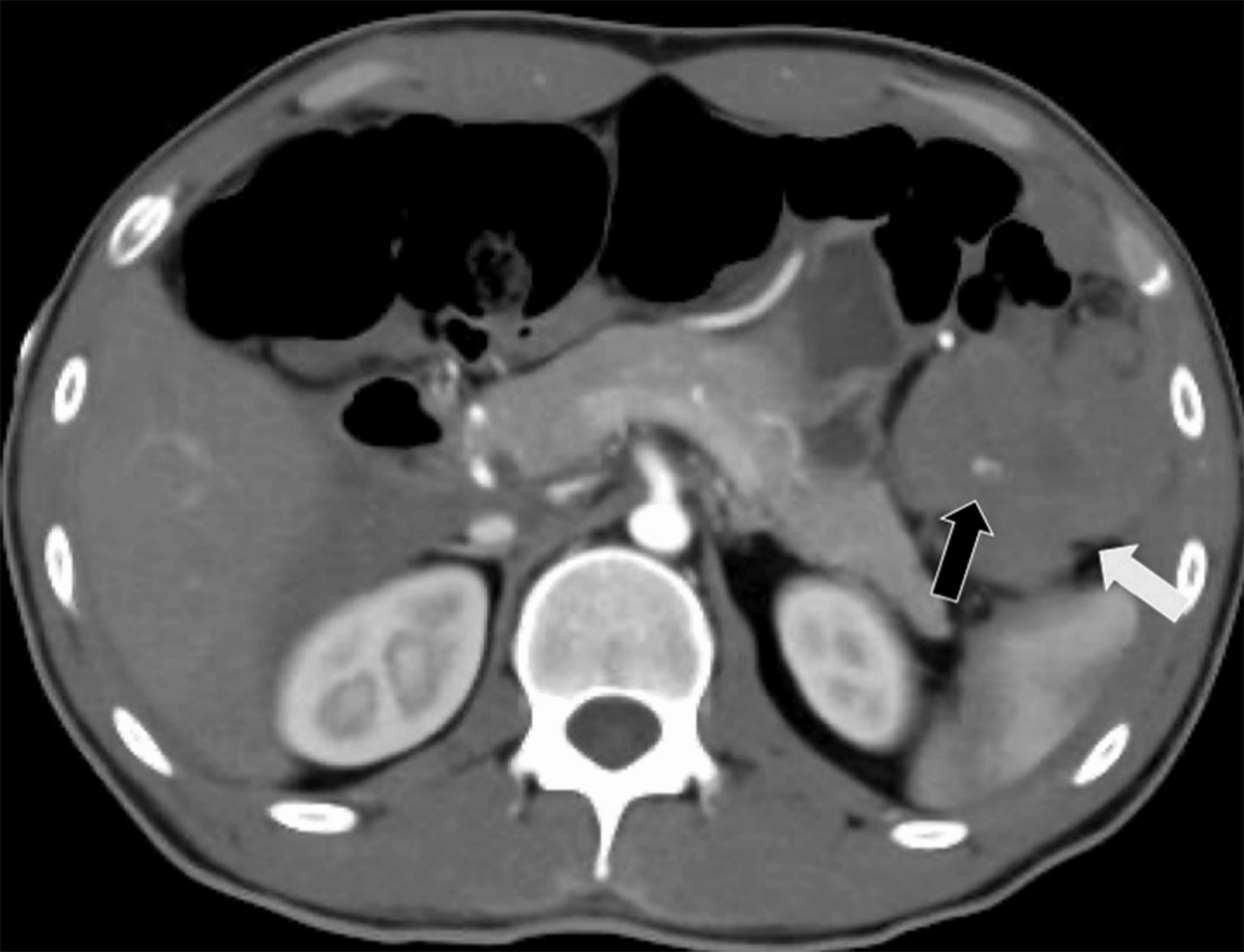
Contrast-enhanced computed tomography reveals intraperitoneal hemorrhage The white arrow indicates a clot around the omentum. The black arrow indicates the extravasation of the contrast media.
